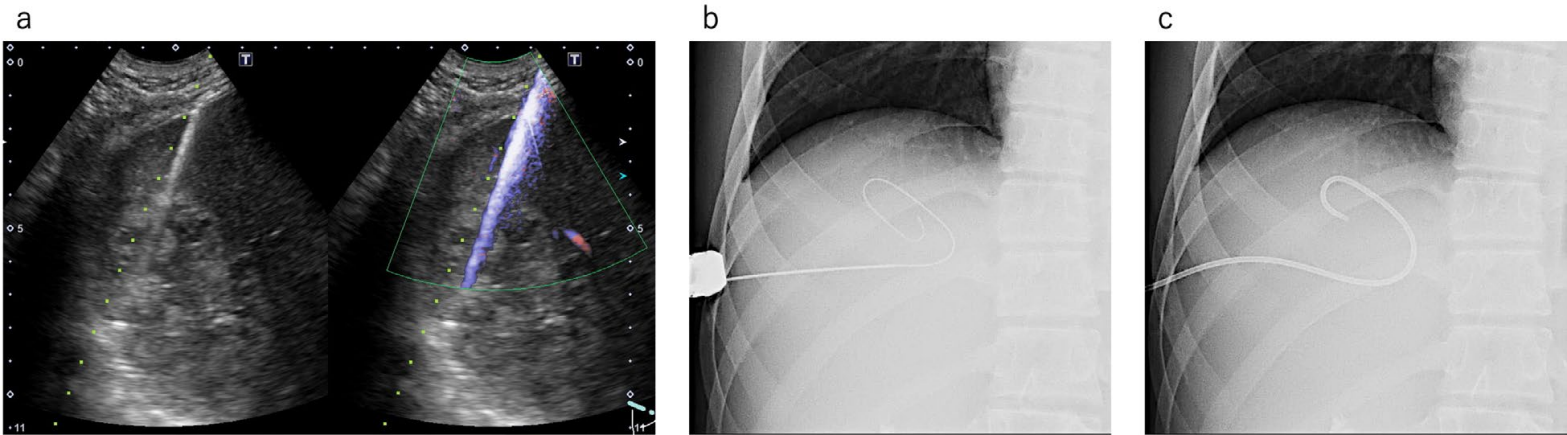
Technical tips for percutaneous transhepatic liver abscess drainage. (a) The liver abscess was punctured using an 18‐G aspiration needle under ultrasound guidance. (b) A 0.035‐inch guidewire is inserted into the cavity of the liver abscess. (c) A pigtail drainage tube is placed within the liver abscess.
